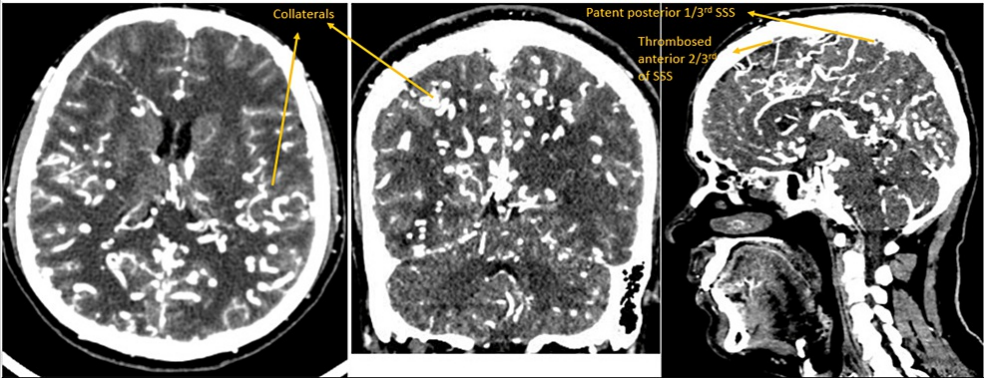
Contrast-enhanced CT head and neck - axial, sagittal, and coronal sections - shows diffuse tortuous cortical, intraparenchymal, and extracranial veins in bilateral cerebral and cerebellar hemispheres suggestive of dural arteriovenous fistula.
